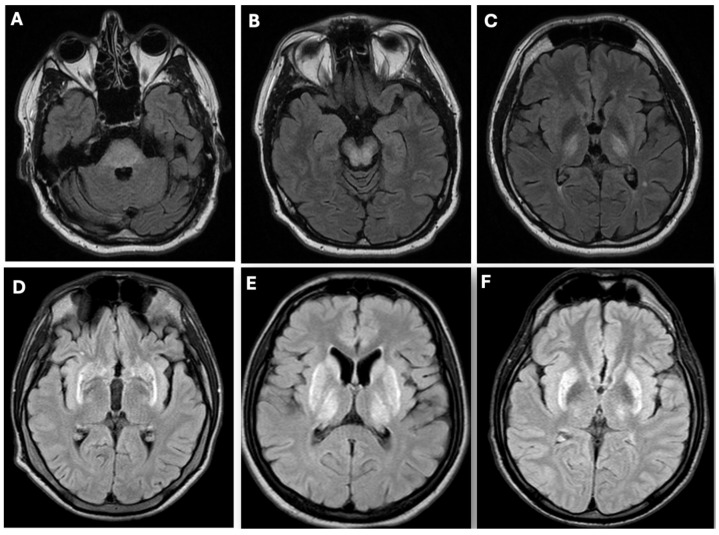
Brain MRI pathology in Wilson’s disease—symmetrical hyperintense signal in FLAIR sequences in: (A) pons, (B) midbrain, (C) putamen and thalamus, (D) putamen, (E) putamen, thalamus and head of nucleus caudate, (F) putamen and thalamus (authors’ material from the Department of Neurology).
